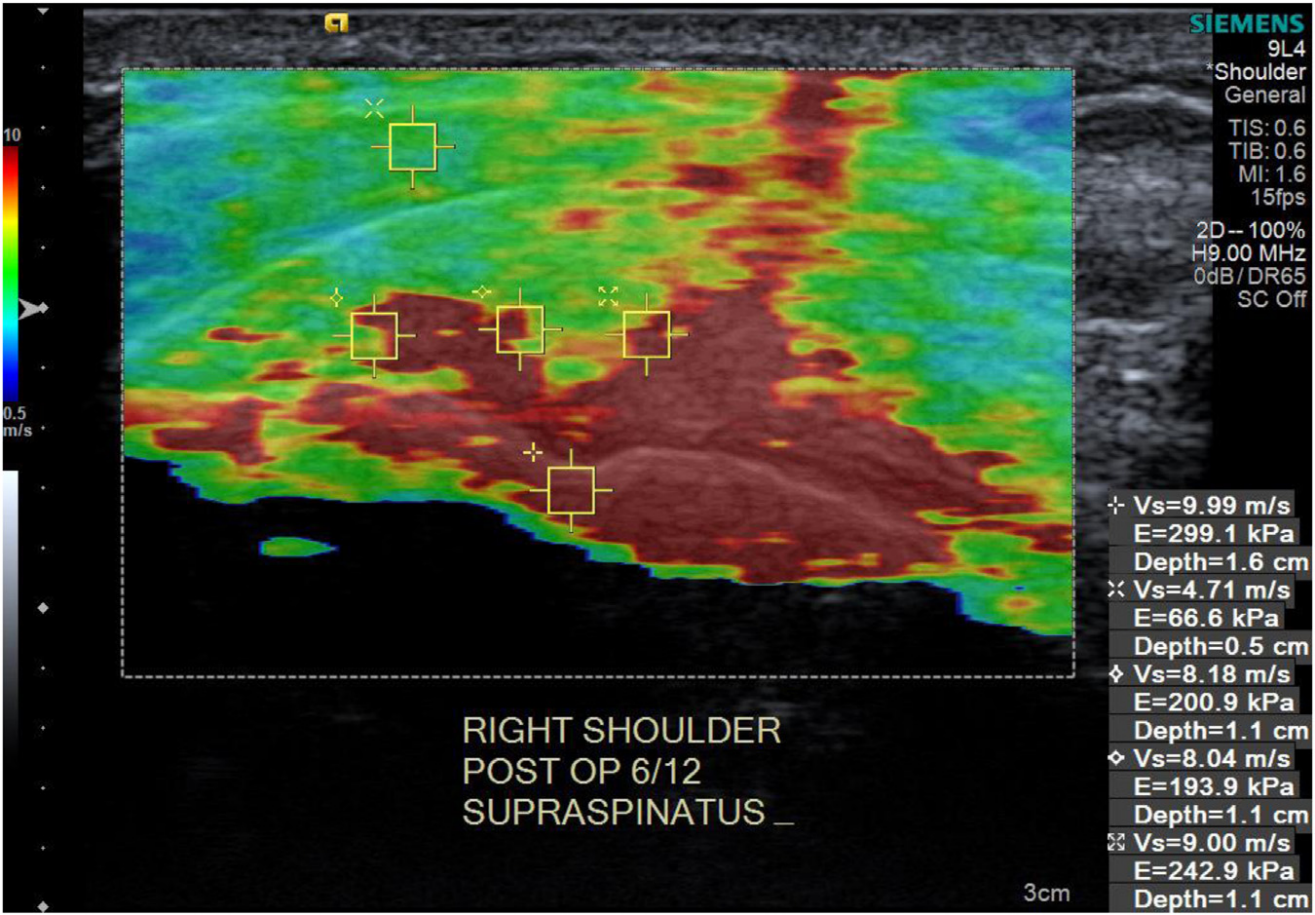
Shear wave elastography ultrasound ‘colour elastogram’ of the right supraspinatus tendon, where stiff tissue appears red and soft tissue appears blue from Hackett et al.^27^
